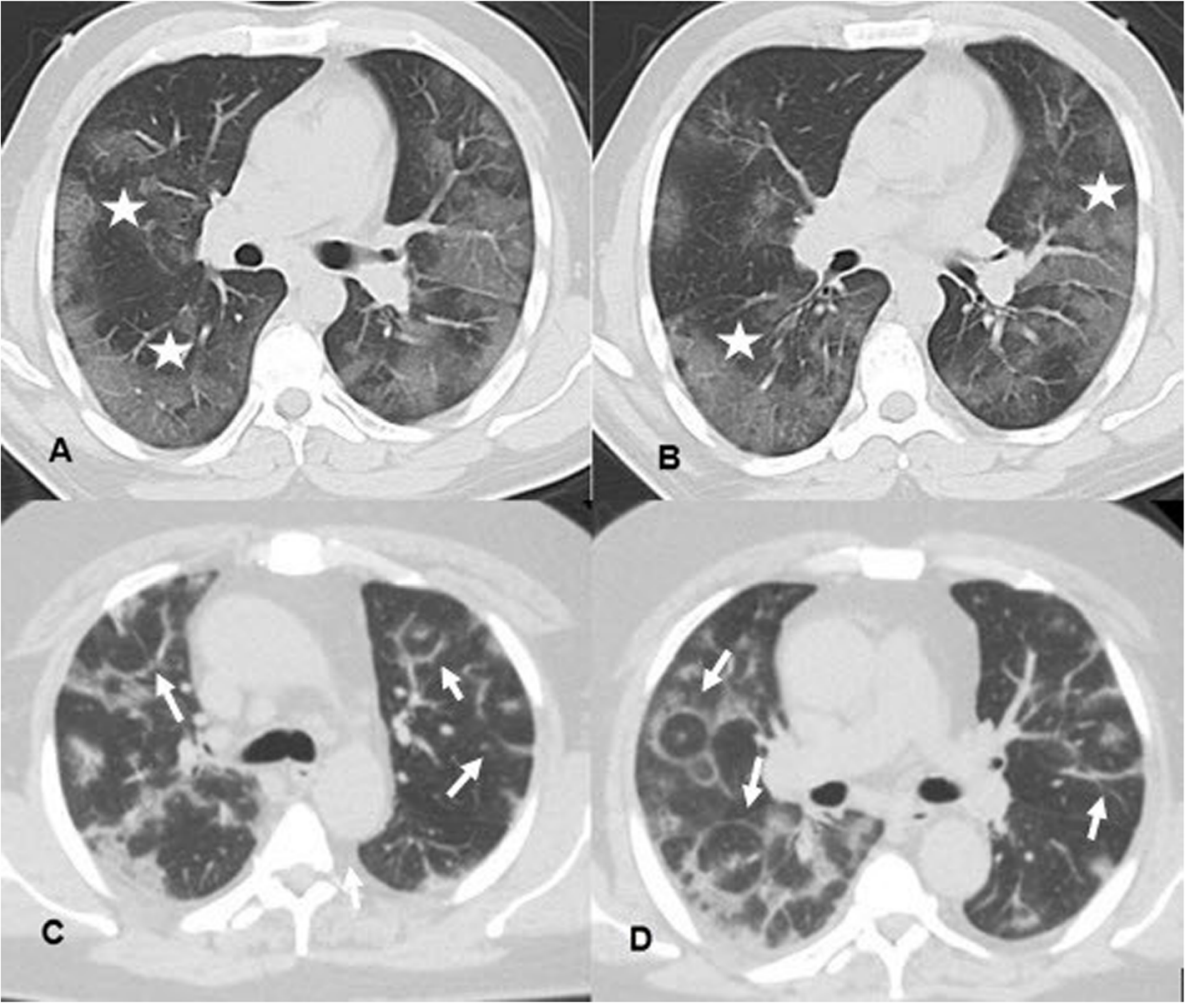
(A and B) Axial CT images, lung window, of a 38-year-old male who complained of fatigue, fever, and cough of 6 days duration. The images revealed bilateral peripheral multifocal ground-glass opacities with interlobular septal thickening giving the crazy paving appearance (white stars). (C and D) Axial CT images, lung window, of a 55-year-old male patient who suffered from 10 days of fever, dyspnea, and cough which revealed multiple reverse halo signs (white arrows) and subsegmental consolidation. Both patients reported as high probability cases for COVID-19 following the RSNA recommendations were RT-PCT positive and were hospitalized
